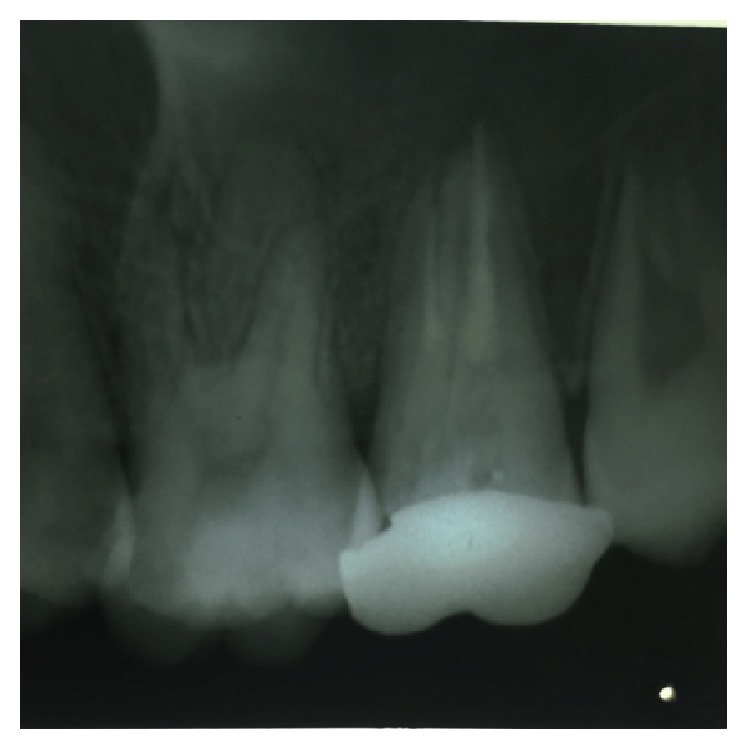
Radiograph showing external reinforcement with metal crown in tooth 27.
